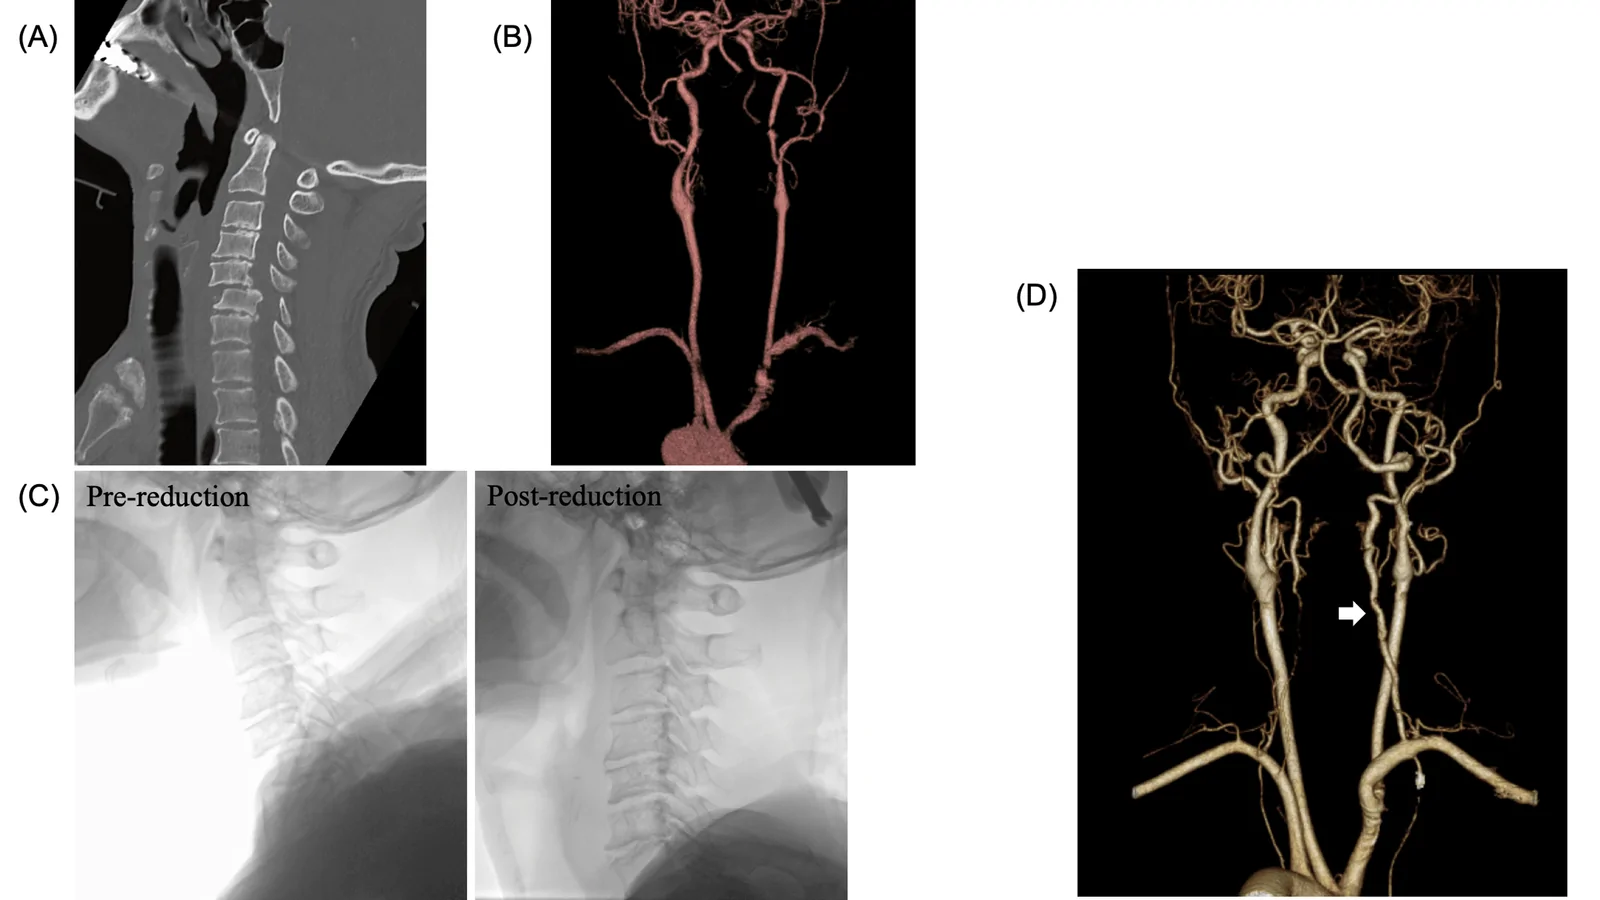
Imaging findings of case one (A) Sagittal CT showing C5-6 cervical fracture-dislocation; (B) Pre-reduction CTA demonstrating bilateral vertebral artery occlusion; (C) Fluoroscopic images obtained before and after awake closed reduction; (D) Follow-up CTA demonstrating recanalization of the right vertebral artery (arrow) CTA: Computed tomography angiography; VAI: Vertebral artery injury
